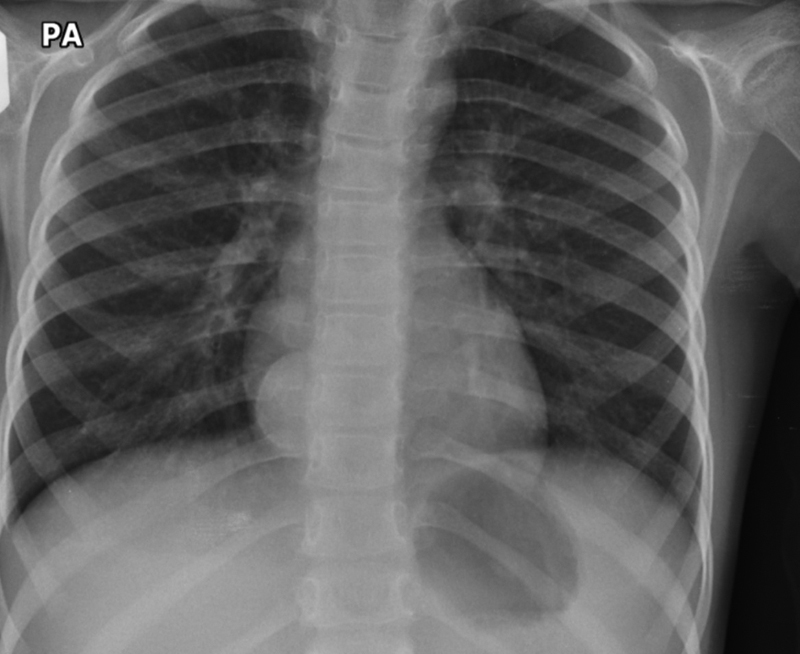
Chest X-ray showing a lesion in the right hemithorax situated within the right atrial shadow.
